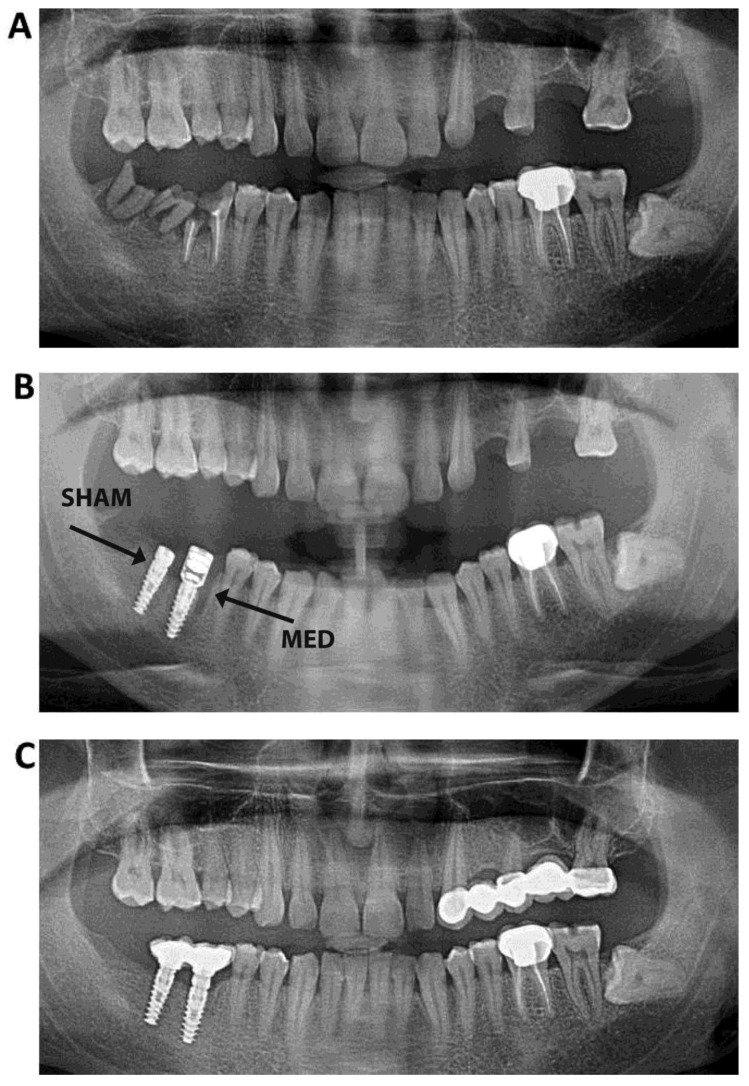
Radiographic appearance of a miniaturized electromagnetic device (MED; test) and sham (control) healing abutments, (A) before the implantation; (B) 6 weeks post-implantation and (C) 12 weeks post-implantation.
